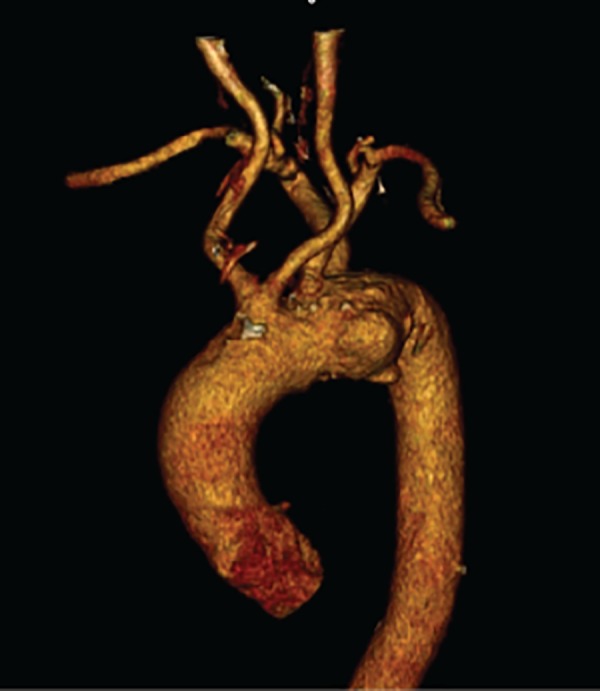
Preoperative CT-A 3D reconstruction
